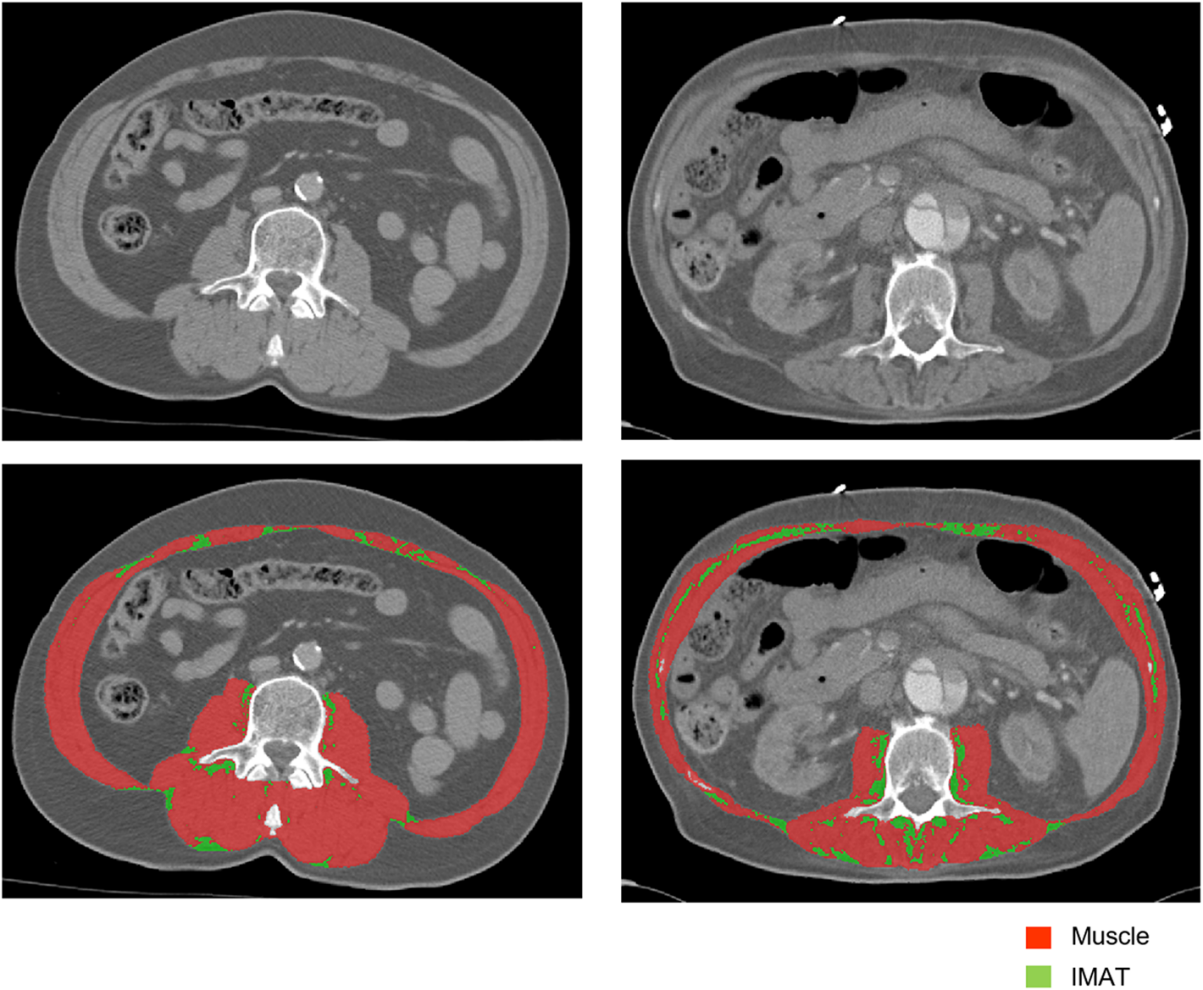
Example of CT scan analysis. This image shows CT scans at the level of lumbar vertebra 3 of two patients both un-analysed (*upper row*) and analysed (*lower row*). The analysed images show muscle tissue (*red*) and intermuscular adipose tissue (*IMAT*, *green*). The patient on the left has more muscle (165 vs. 120 cm^2^), less IMAT (10 vs. 19.5 cm^2^), and higher mean skeletal muscle density (42 vs. 18 Hounsfield Units) than the patient on the right
